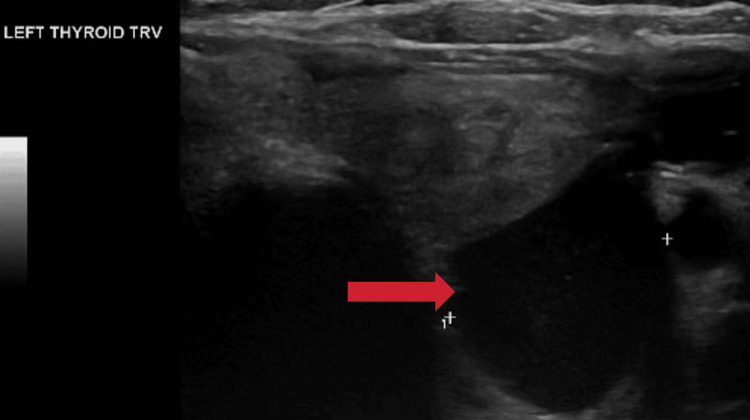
Transverse thyroid US showing a 2.4 x 2.0 x 4.4 cm hypoechoic cystic mass located posterior to the left inferior thyroid pole as indicated by the red arrow US: Ultrasound
